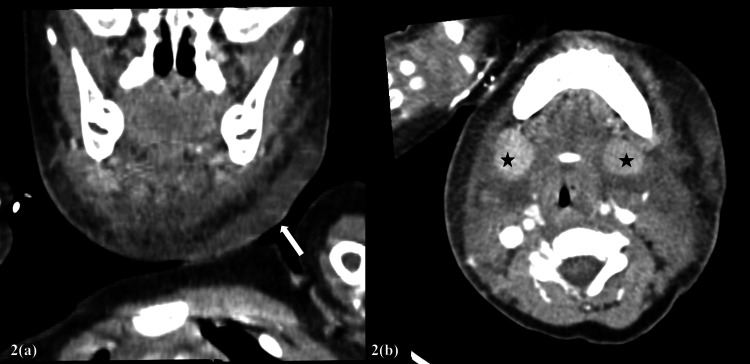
CECT of the neck (a) Features of Ludwig's angina (white arrow), with no evidence of an abscess; and (b) bilateral submandibular sialadenitis (black star) CECT: contrast-enhanced computed tomography
